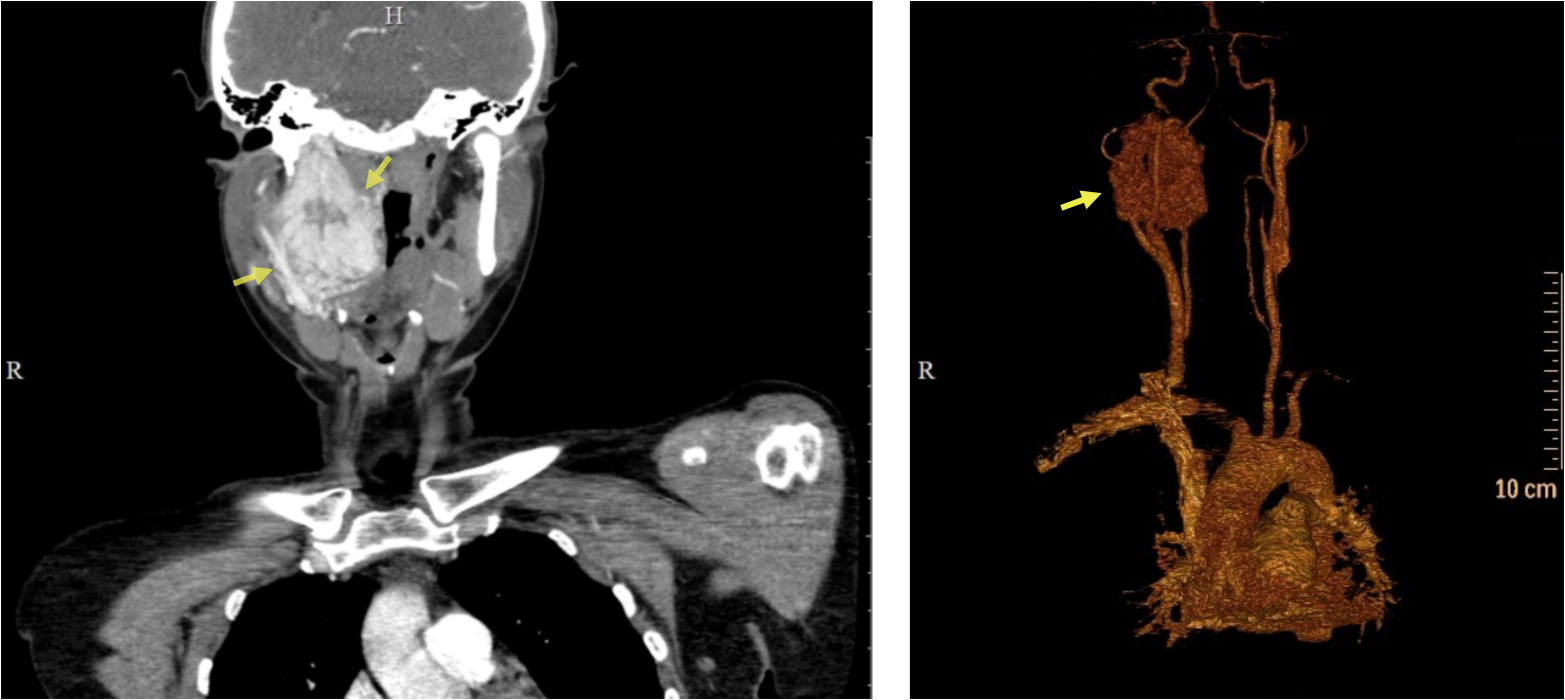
Preoperative computed tomography of the patient’s head and neck. CT scan (left), 3D reconstruction (right). Yellow arrows indicate the tumor.
